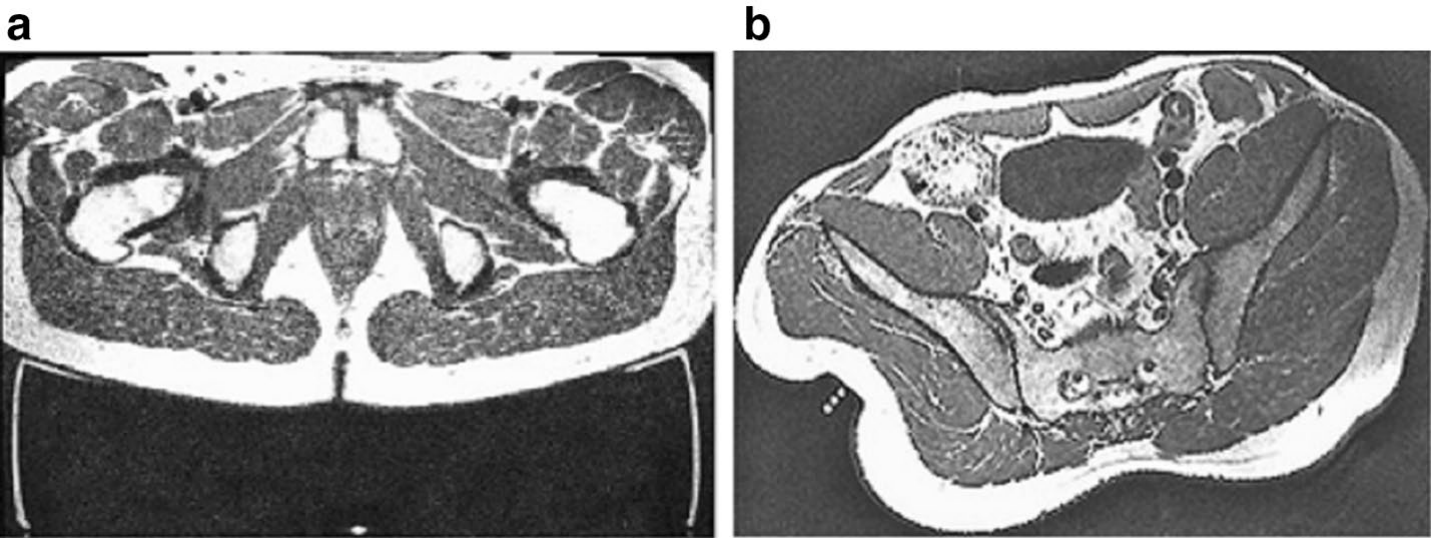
a MRI of seated buttocks and b gluteal muscle subjected to maximum indenter displacement with highlighted markers [146]
